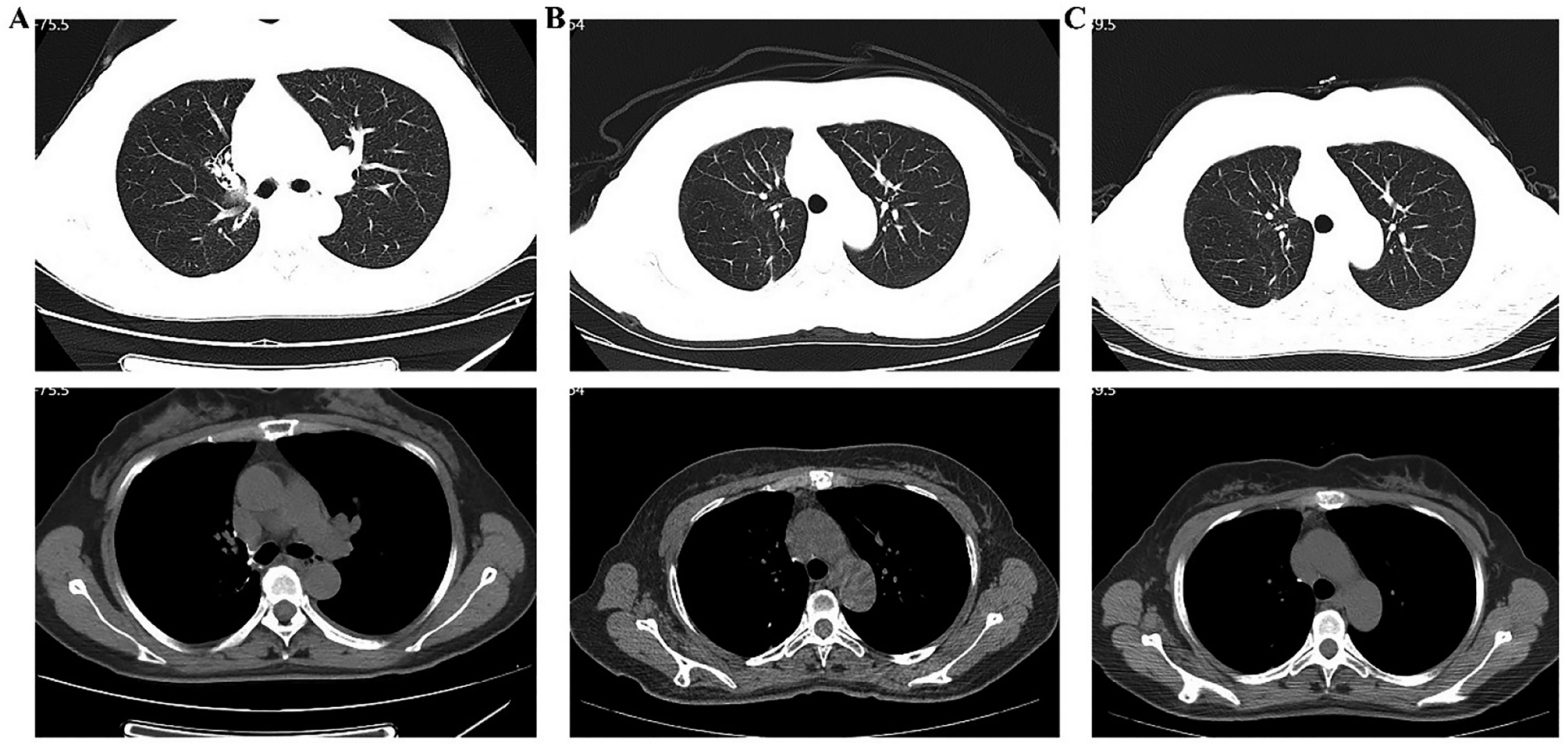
Chest CT scans showing postoperative changes in the right upper lung. (A) July 30, 2024; (B) January 6, 2025; (C) July 24, 2025.
